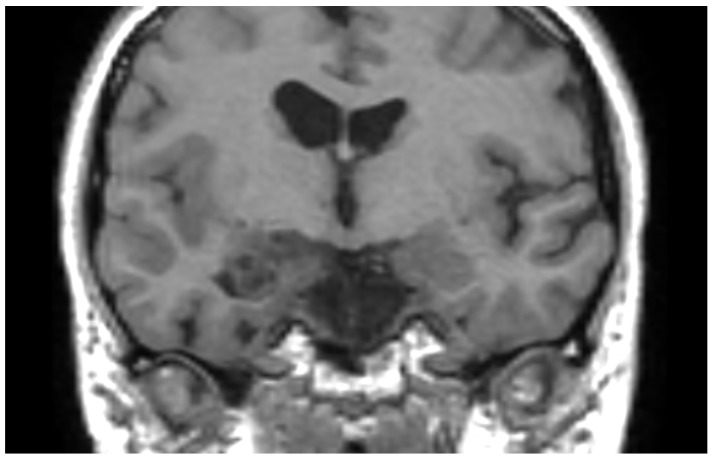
Coronal non-contrast T1-weighted MRI of the patient’s right hippocampus DNET at presentation. There is no evidence of the medially located lesion provoking midline shift or surrounding edema.
